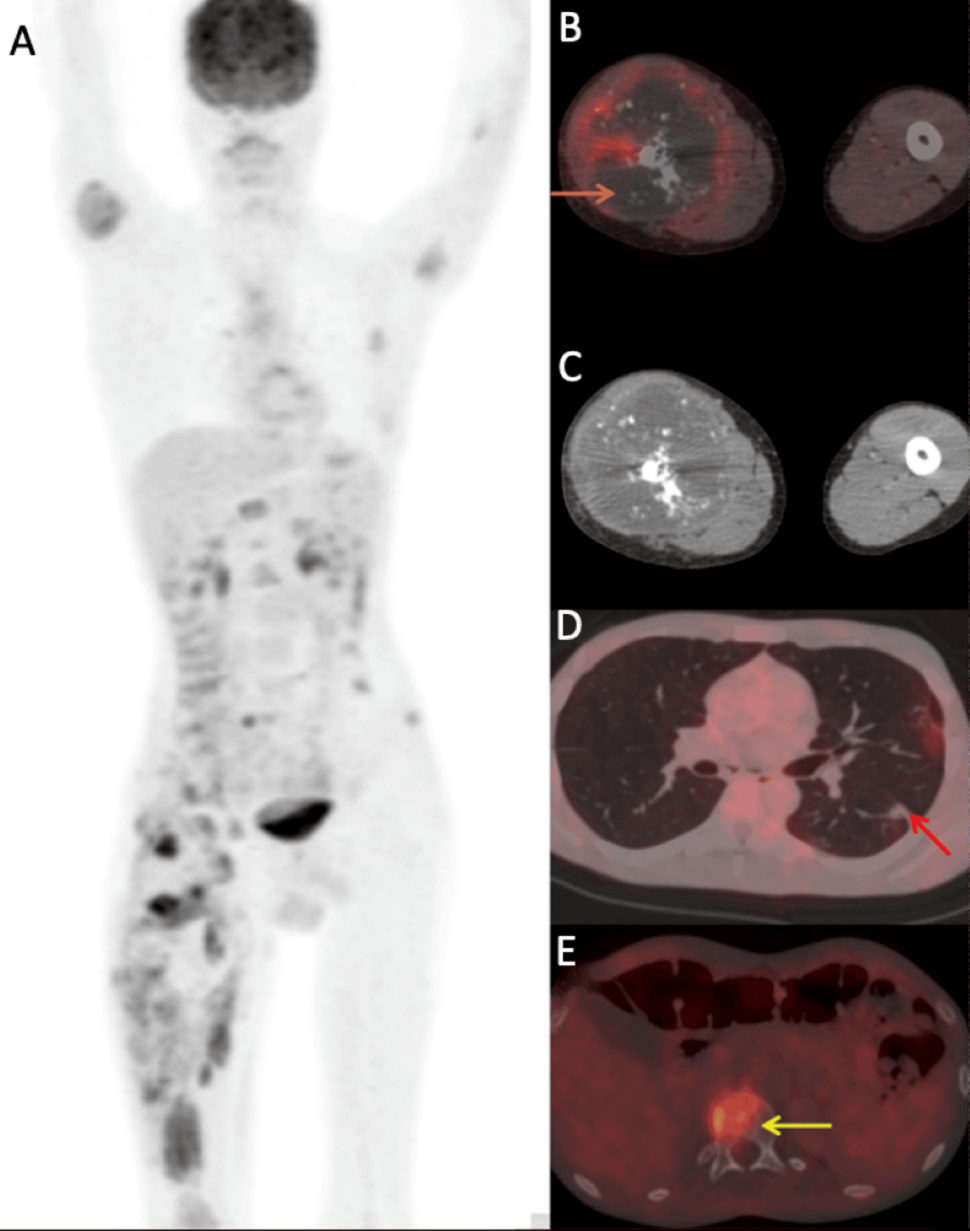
Follow-up F-18 FDG PET-CT images Follow-up F-18 fluorodeoxyglucose (FDG) Positron Emission Tomography (PET)/Computed Tomography (CT) images (maximum intensity projection (MIP) – A, fused axial PET-CT – B, D & E, axial CT – C) for response assessment; on cabozantinib, showing interval reduction in FDG avidity and size of primary mass involving right gluteal and right thigh muscles (orange arrow), peri-fissural left lung lower lobe nodule (red arrow) and skeletal lesions (yellow arrow); suggestive of partial response to therapy.
